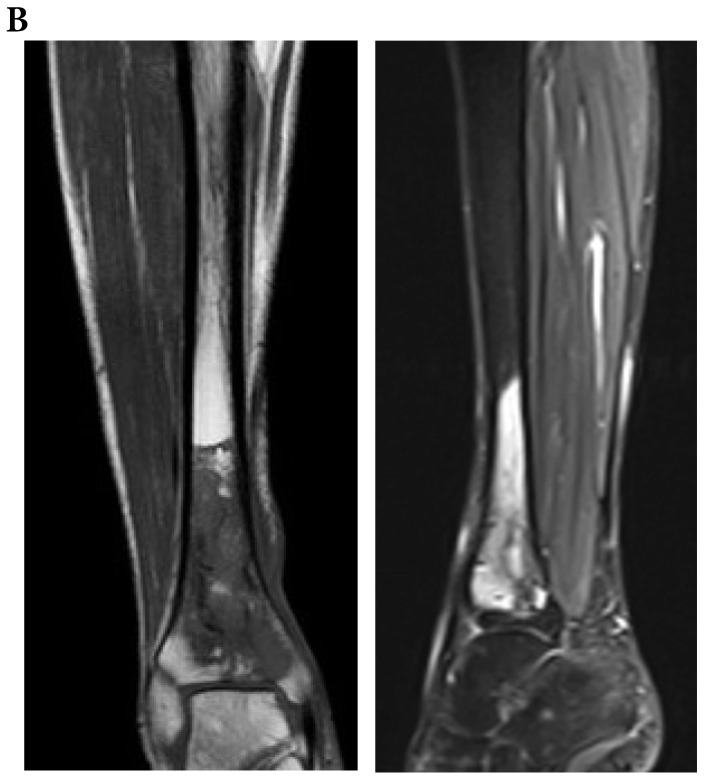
Pre-operative radiograph (a) and MRI (b) suggestive of Cierny and Mader Stage 3 osteomyelitis of the lower tibia.
